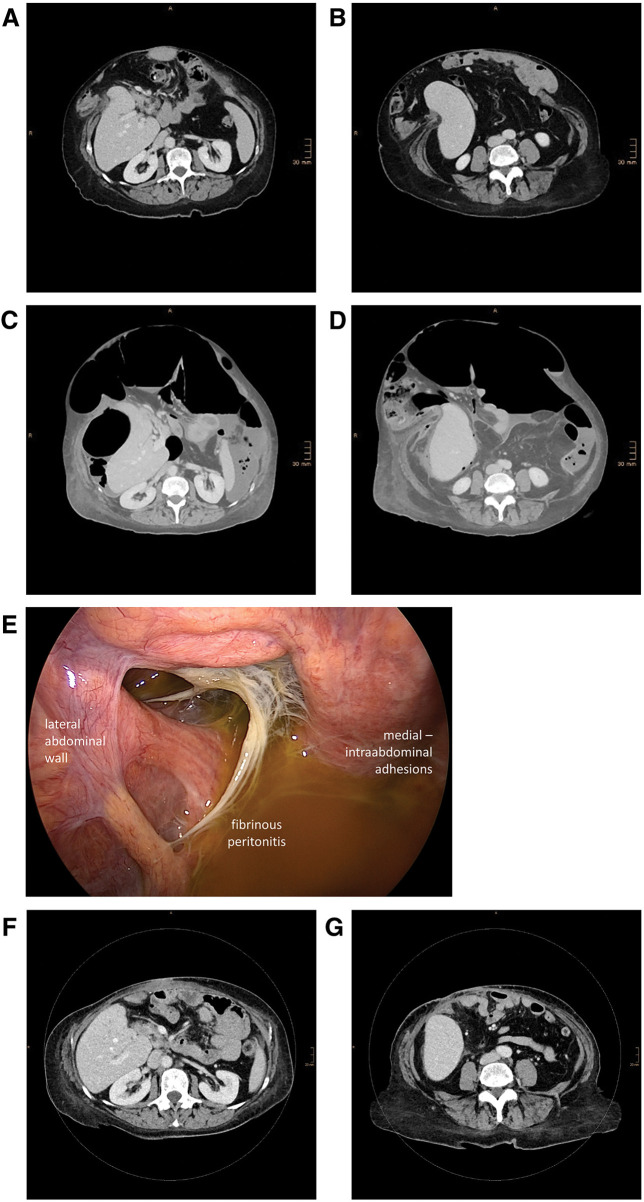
Preinterventional, preoperative, intraoperative, and postoperative aspects of the complex recurrent incisional hernia. (A, C, and F are on the same level (lumbar vertebra L2–hilum of kidneys) and B, D, and G are on the same level (lumbar vertebra L4–caudal edge of kidneys)). Preinterventional: (A and B) Preinterventional CT scan of the recurrent incisional hernia with 47% loss-of-domain prior to the chemical component separation and establishment of the PPP. Preoperative: (C and D) CT scan performed preoperatively due to increased inflammatory markers. The CT scan shows the iatrogenic PPP and perihepatic and perisplenic fluid collections. Intraoperative: (E) Laparoscopic view of the right upper quadrant. Fibrinous peritonitis with ascites can be detected. Postoperative: (F and G) 7-month postoperative CT scan. Complete restoration of the abdominal wall integrity after Rives-Stoppa-Sublay-Herniotomy with bilateral component separation is shown in the scan. No signs of recurrence or fluid collections/mesh infection are present.
